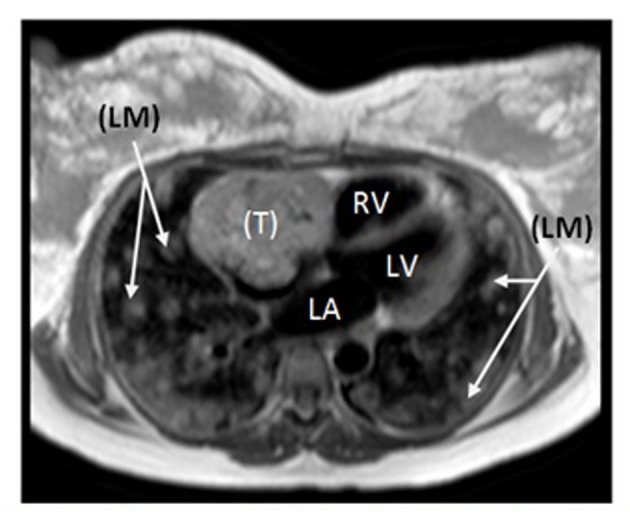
Cardiac MRI revealing large irregular tumor (T) occupying most of the RA, with numerous bilateral lung metastases (LM). RV: right ventricle; LA: left atrium; LV: left ventricle.
